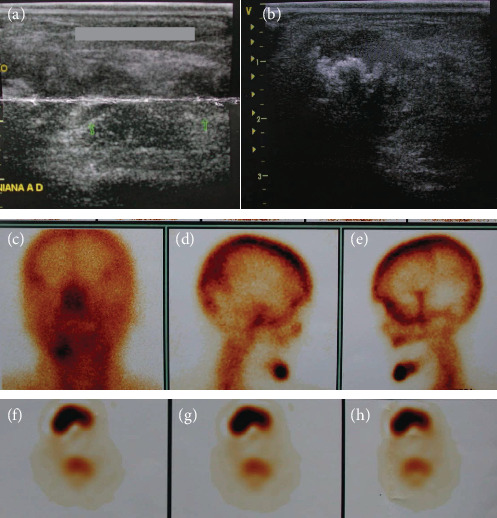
(a, b) Ultrasound image of the submental region with heterogeneous and hyperechoic areas, with acoustic shadowing and thickening compatible with swelling. (c–h) Scintigram shows marked uptake in the right side of the mandible.
